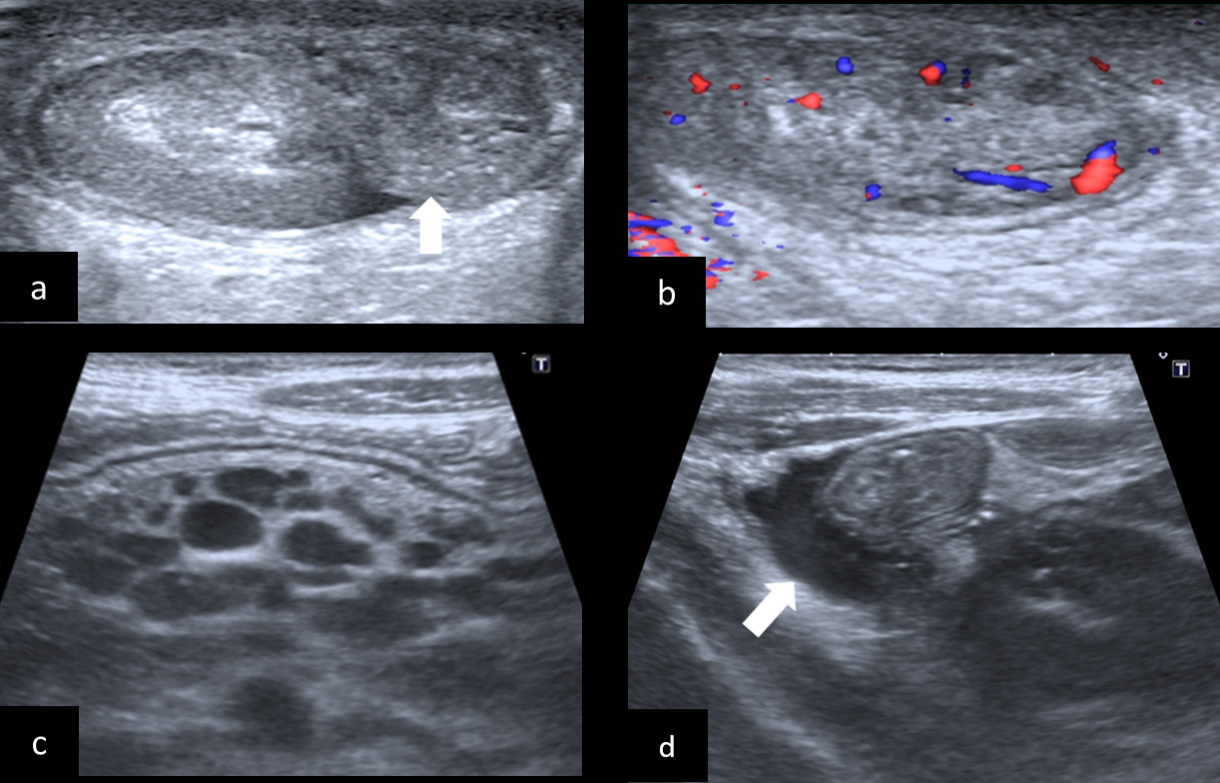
Imaging findings of an 8‐year‐old boy who presented with fever, scrotal pain and swelling. (a) Sagittal plane color Doppler and (b) Grayscale ultrasonography images demonstrate enlargement (white arrow) and hypervascularity of the epididymis consistent with epididymitis. (c) Abdominal ultrasonography of the same patient shows multiple mesenteric lymph nodes with mesenteric inflammation in the right lower quadrant and (d). small-volume ascites (white arrow).
